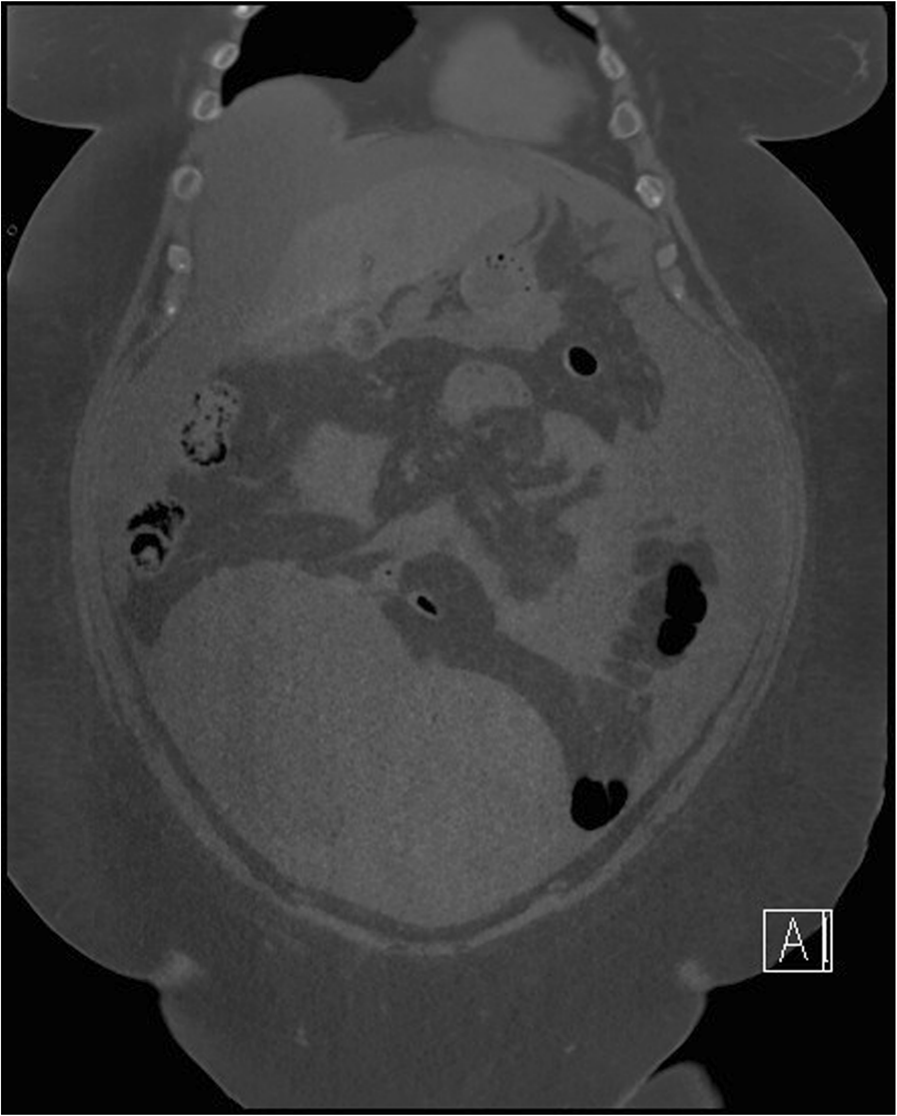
Non-contrast abdominal computed tomography of the patient revealing the 18-cm large ovarian lesion with moderate volume peritoneal ascites
